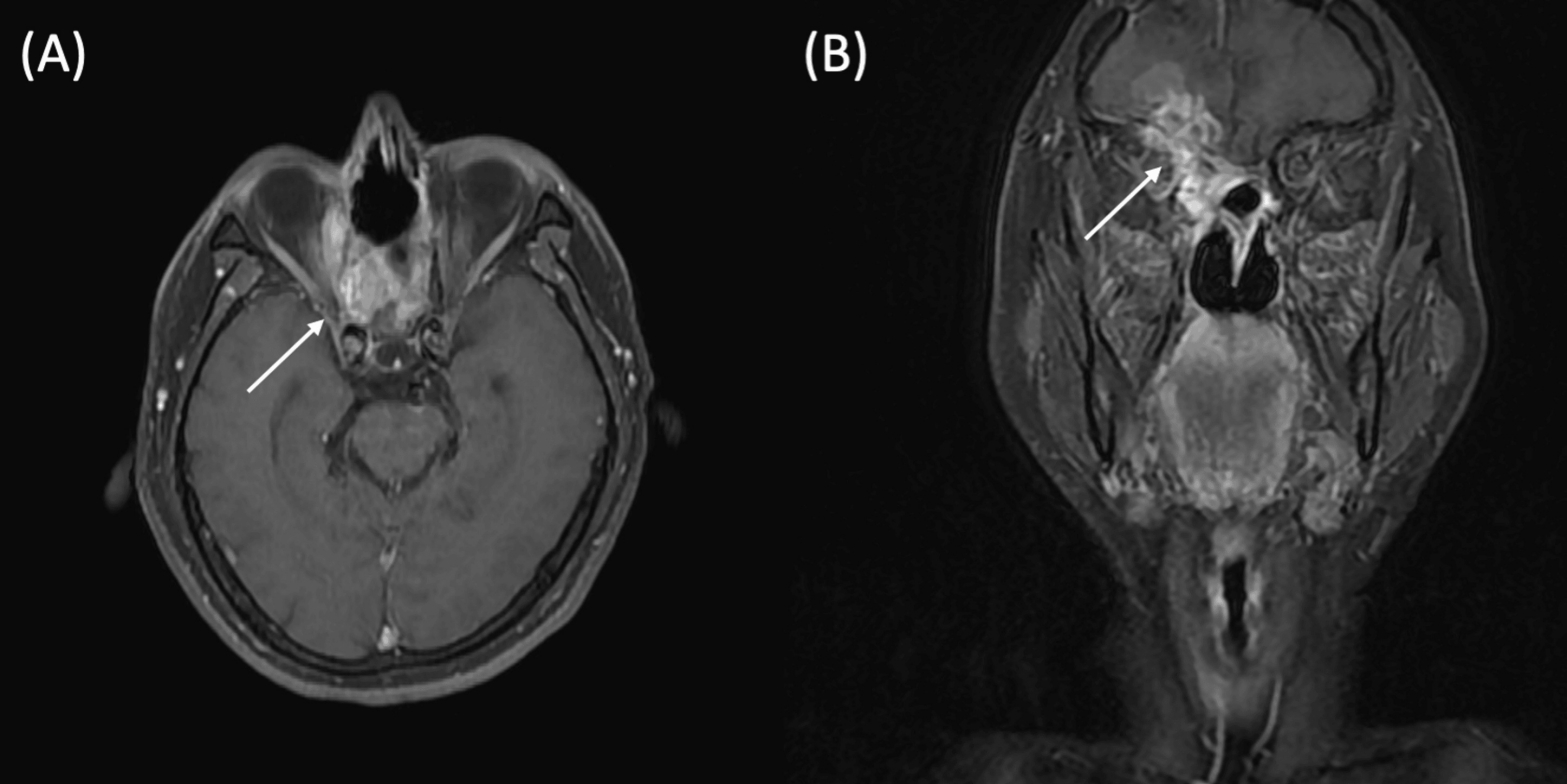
Axial and coronal view of contrast-enhanced T1 MRI eight months post-1st BNCT. (A) Axial view and (B) Coronal view obtained eight months after the first BNCT course. White arrows indicate the location of the recurrent tumor mass and post-BNCT cystic change. BNCT: boron neutron capture therapy.
